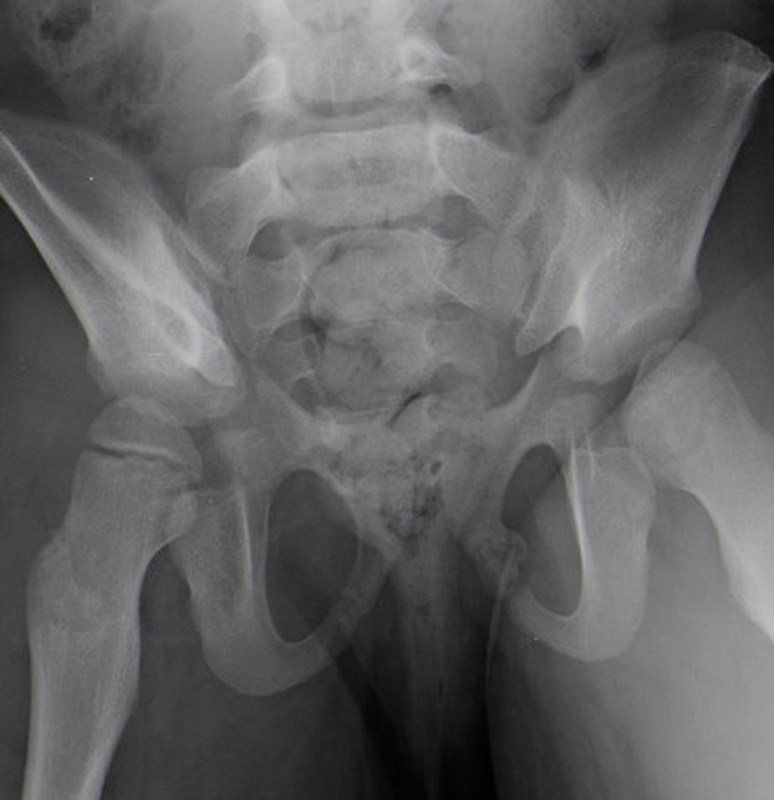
Radiography of the pelvis (outlet view).
